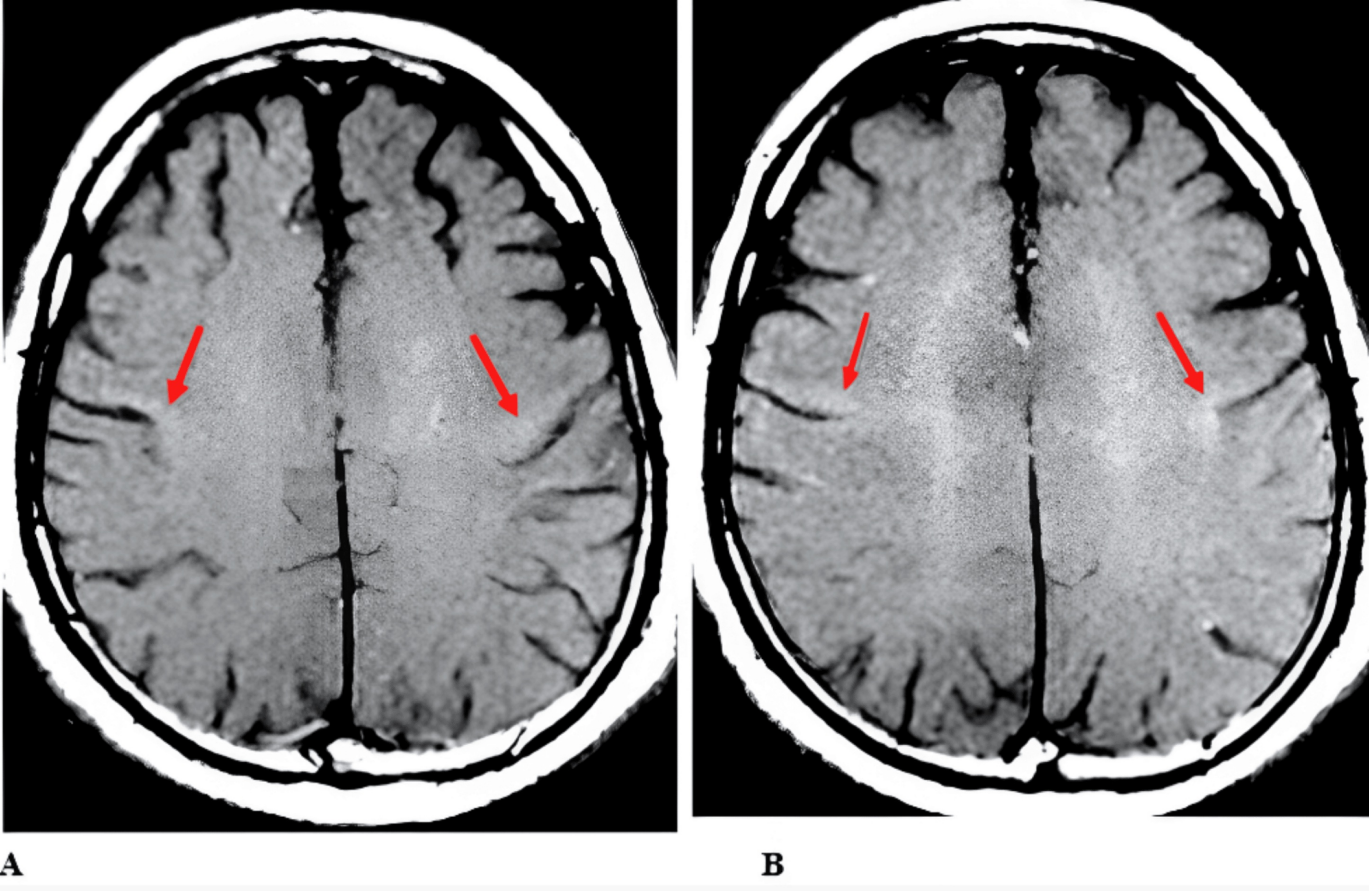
T1-weighted sequence with magnetization transfer technique showing a subtle hypersignal along the pyramidal pathway (arrows in A and B)
